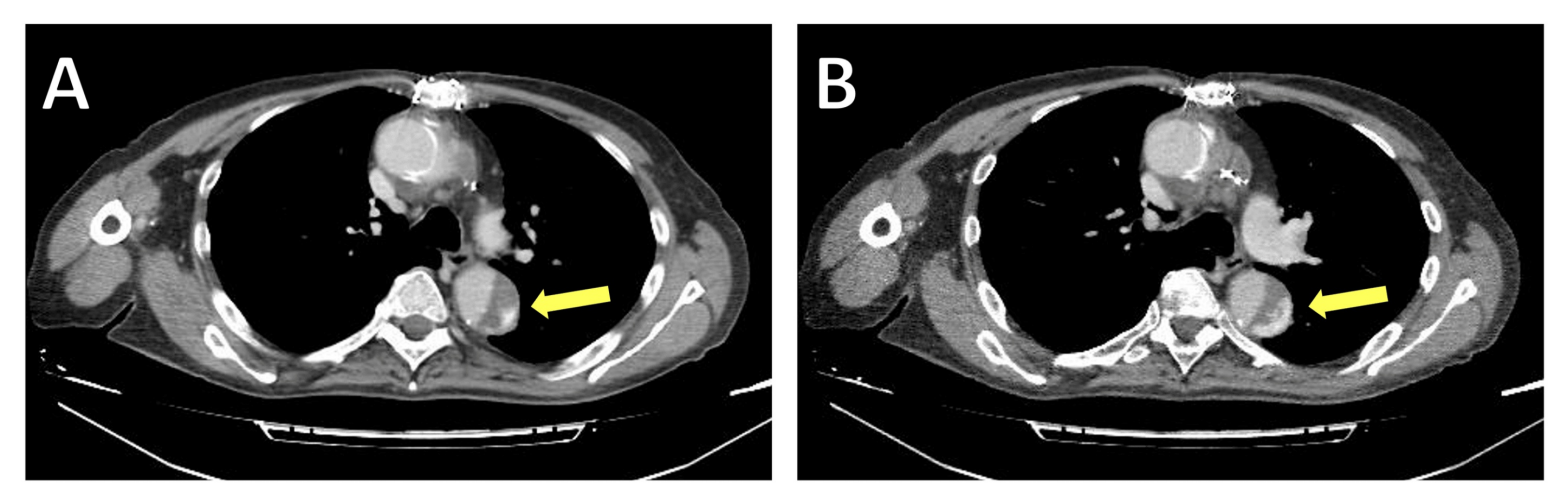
Follow-up chest CT findings: (A) First-month follow-up showing no pleural effusion and chronic dissection of the descending aorta, partially thrombosed (arrows), without significant dilatation. (B) Sixth-month follow-up confirming stability, with no pleural effusion and unchanged chronic dissection of the descending aorta (arrows).
